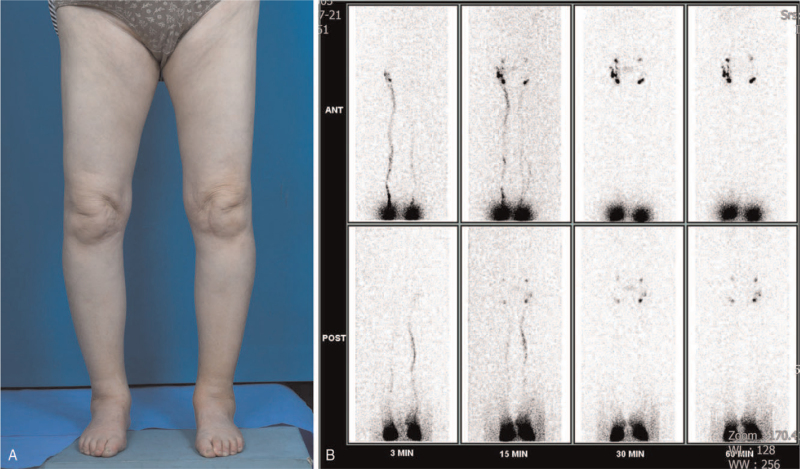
Clinical photograph of patient. (A) A 79-year-old woman manifested bilateral lower extremity swelling 1 week after COVID-19 vaccination. (B) Lymphoscintigraphy showed a decreased lymphatic transport in both lower extremities, especially on the left side.
